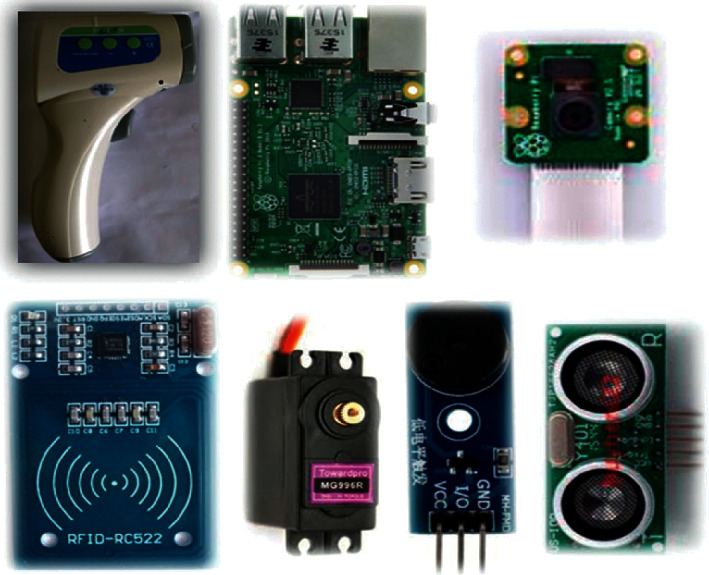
Components of the autothermometer system (from top left): a forehead thermometer, a Raspberry Pi device, a camera module, an RFID reader, a servo motor, a buzzer, and an ultrasonic module.
